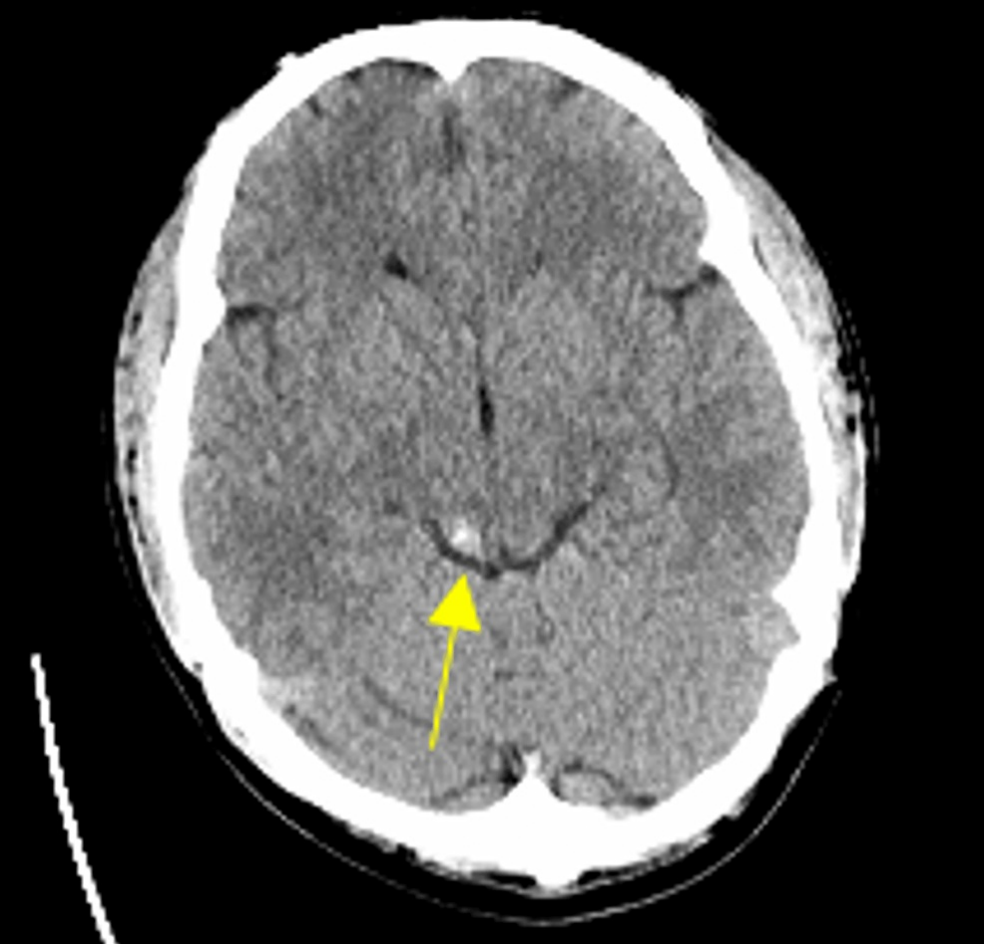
CT of the head at the onset of symptoms A 5 mm hyperdensity in the right posterior midbrain is shown.
